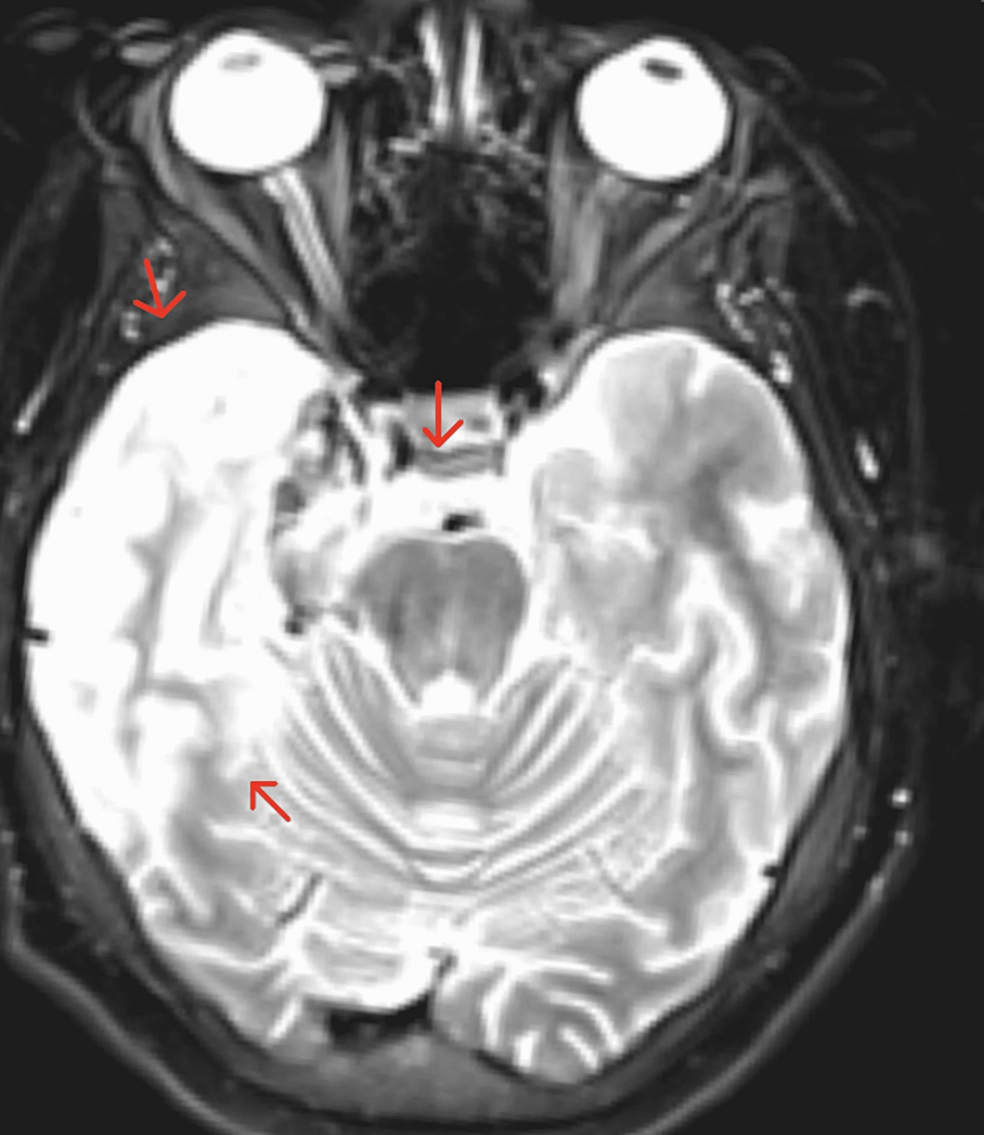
MRI brain with contrast in a T2-weighted axial view The axial view shows contrast hemorrhagic transformation with a signal alteration involving the right limbic system, right fornix, and right optic radiation. Mild effacement of the right lateral ventricle and minimal leftward midline shift.
